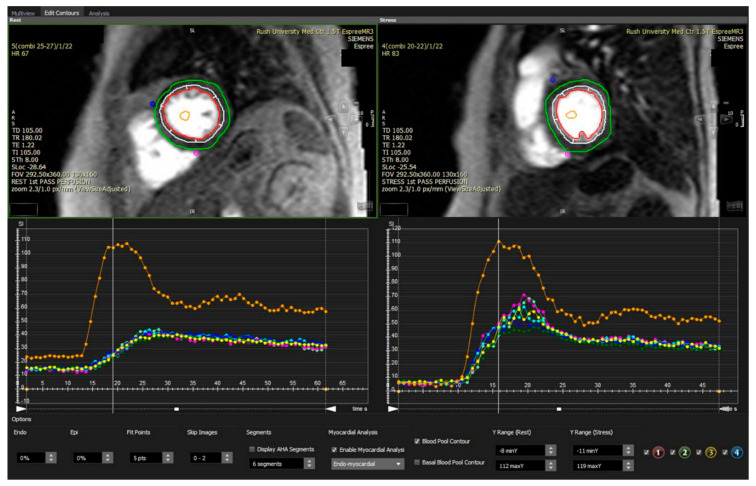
Stress CMR (Cardiovascular Magnetic Resonance) perfusion in a 67-year-old woman with angina and negative coronary CTA (computed tomography angiography) that had shown minimal calcific plaque with <10% stenosis in the proximal left anterior descending artery. After regadenoson stress, no visual reversible perfusion defect was noted, indicating the absence of ischemia from epicardial stenosis; however, the MPRI (myocardial perfusion reserve index) is computed at 1.97, consistent with the diagnosis of CMD (coronary microvascular dysfunction). She was initiated on amlodipine, a high intensity statin and long-acting nitrate therapy and had resolution of anginal symptoms after 2 weeks. In the top panel, Green contours depict epicardium of the left ventricle, Red depicts the endocardial border, and White is the mid myocardium. In the bottom panel, the Orange curve is the signal intensity of blood pool in the left ventricle plotted against time—during Stress regadenoson imaging on the right, and at Rest on the left. The other colored curves depict various segments of the left ventricular myocardium.
